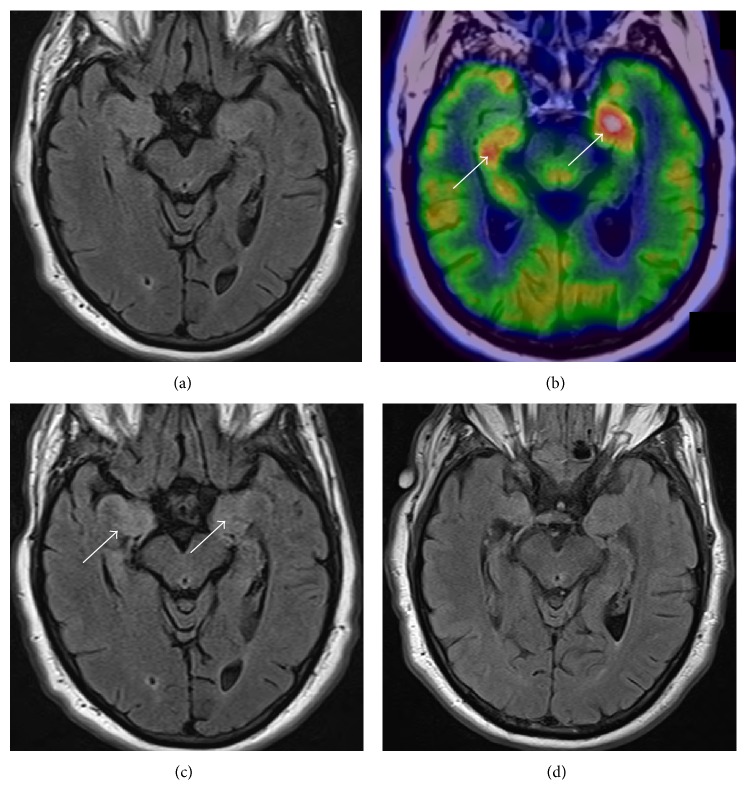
Neuroimaging of VGKC antibody. The initial, unremarkable axial T2/FLAIR MRI of the brain (a) followed by FDG-PET showing significant asymmetric hypermetabolism of the bilateral mesial temporal lobes (arrows; (b)) with repeat axial T2/FLAIR demonstrating hyperintensity in bilateral mesial temporal lobes (arrows; (c)) that significantly improved by the last T2/FLAIR (d).
